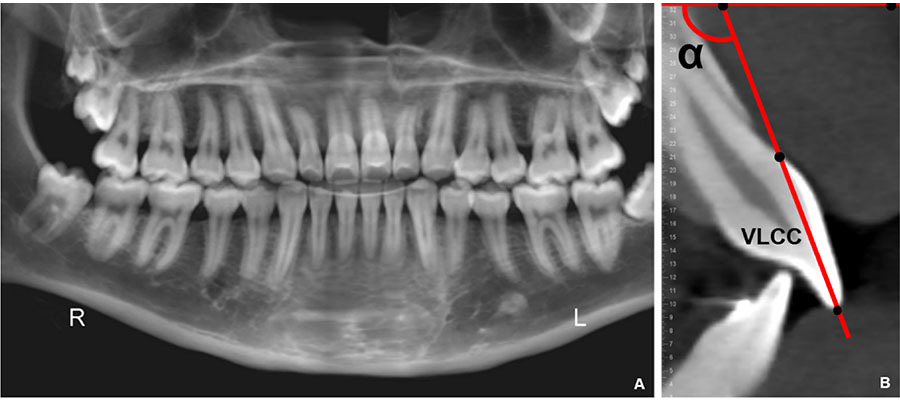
Buccolingual inclination measurements. CBCT-panoramic reconstruction (A); angle formed by the buccal line of the clinical crown (BLCC) until the upper edge of the selected image (B).
